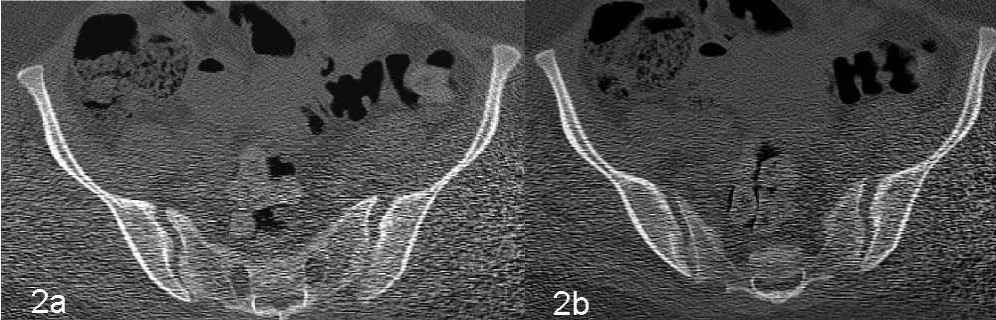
CT scan showing widening of bilateral sacroiliac joints with a slight subluxation of left sacroiliac joint.
